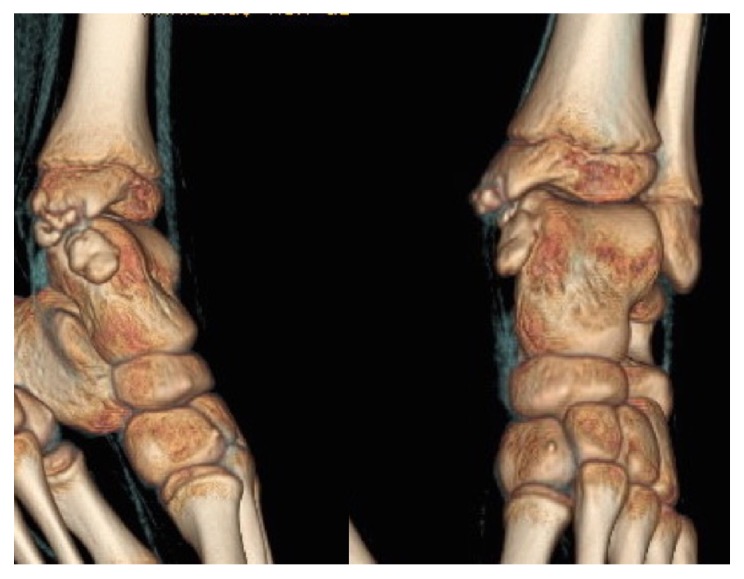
3D reconstruction CT scan showing medial lobulated bony prominences.
